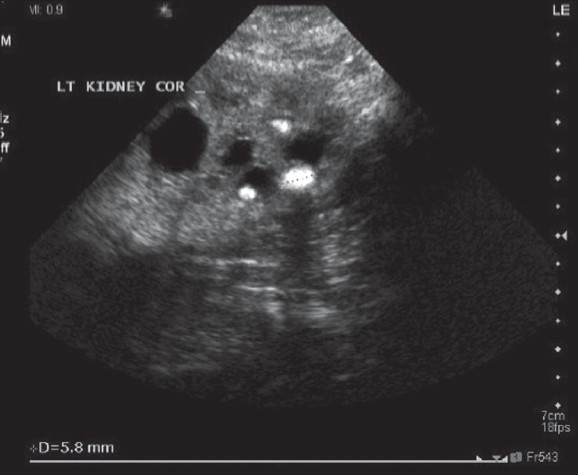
Three-Month-Old Child with Preoperative Renal Calculi on Ultrasound. Ureterorenoscopy Revealed Calcified Papillae
